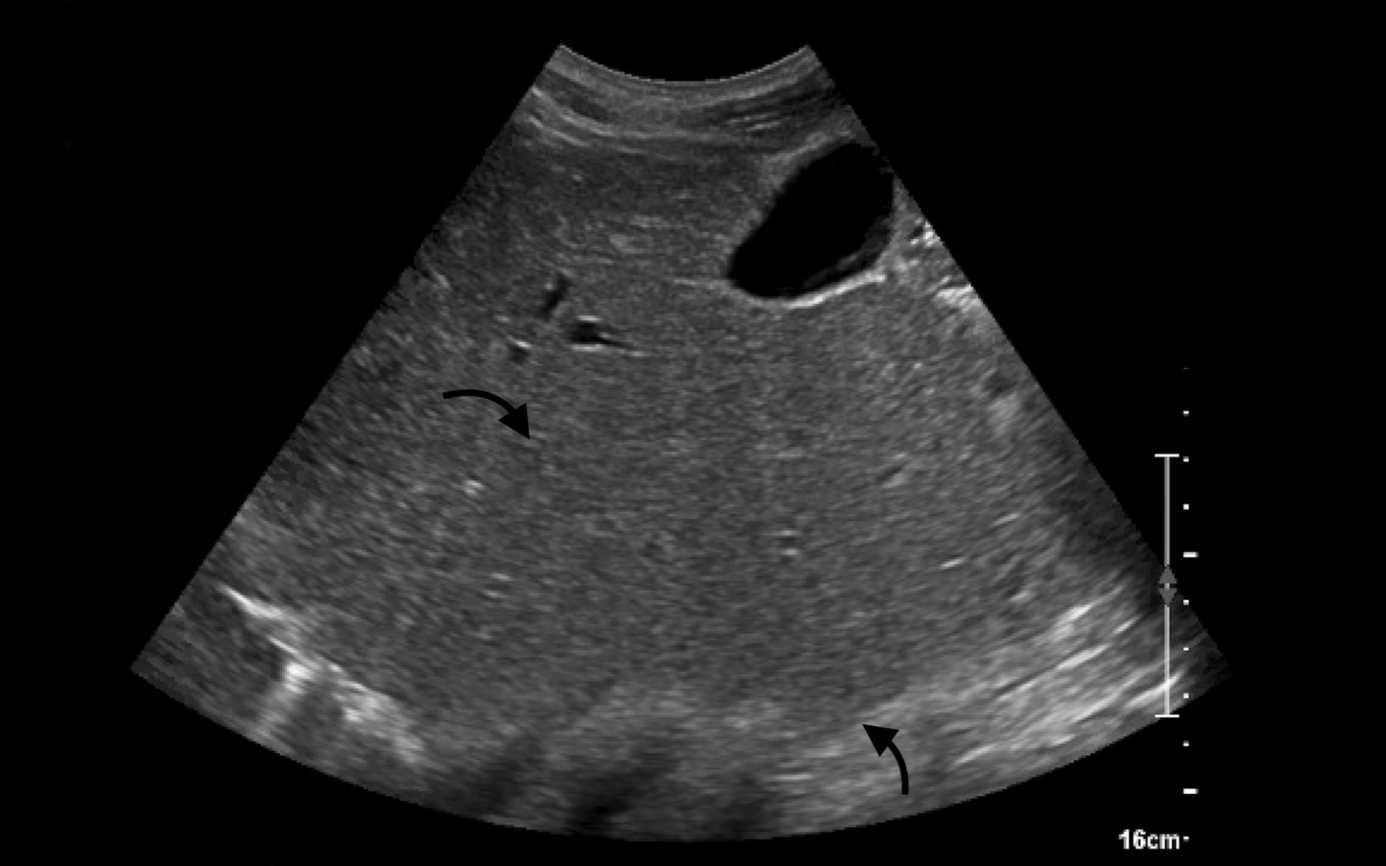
Ultrasound of the Liver Mild coarsened echotexture without evident nodularity, architectural distortion, masses, or intrahepatic biliary ductal dilation.
